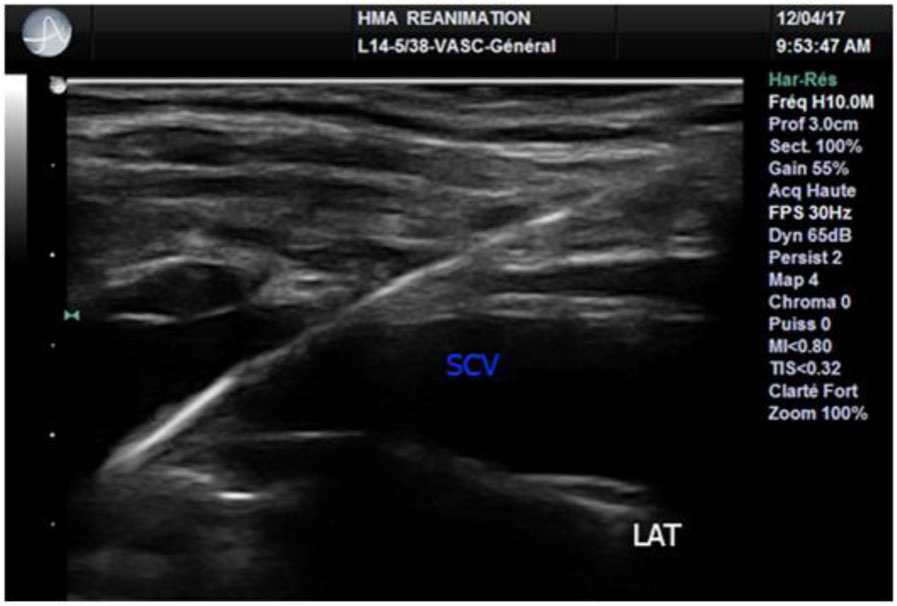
Ultrasound image of the subclavian vein after cannulation and insertion of the guidewire
